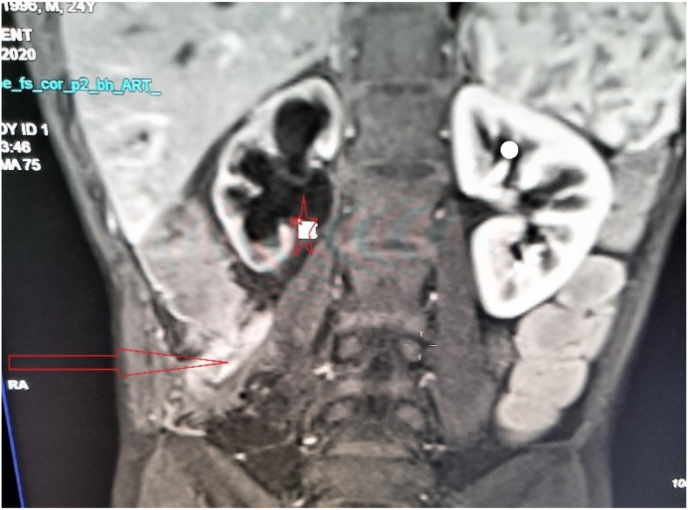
ENTERO-MRI image showing a retroperitoneal collection in the right flank (arrow) with right ureterohydronephrosis (star).
